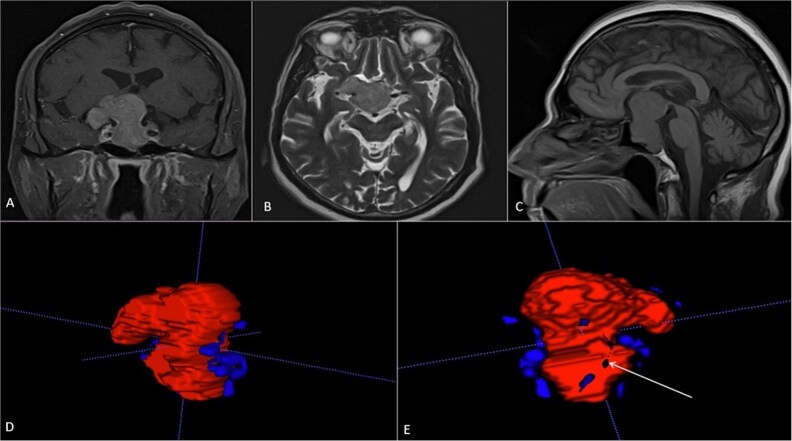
(A, B and C) MRI of pituitary marcoadenoma in coronal, axial and sagittal sections, contrasted T1, T2, and T1 sequences. (D and E) Planimetric segmentation with 3D reconstruction of the basilar and carotid system. White arrow, relationship of the dorsum of the tumor with the basilar artery.
